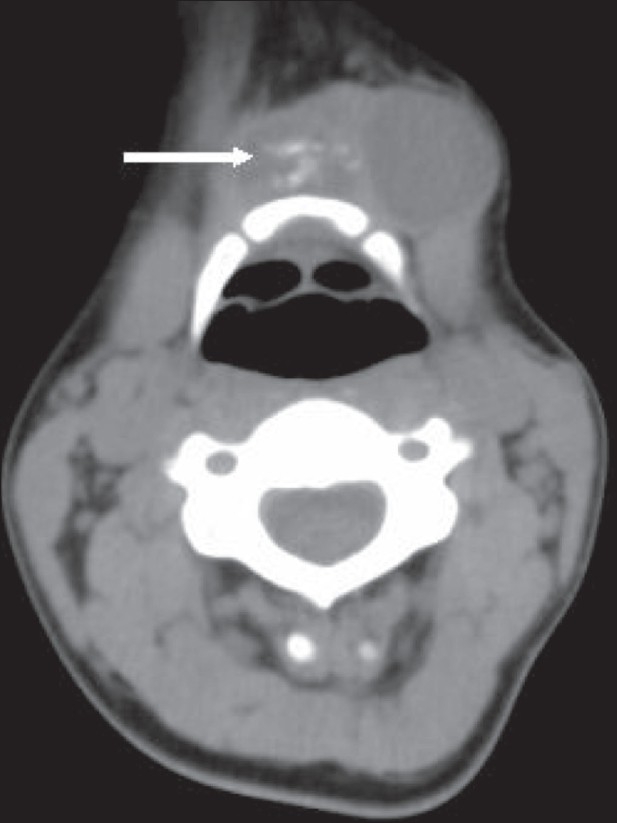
Axial non-contrast-enhanced CT scan at the level of the hyoid bone shows a multicystic lesion with calcification (arrow)
